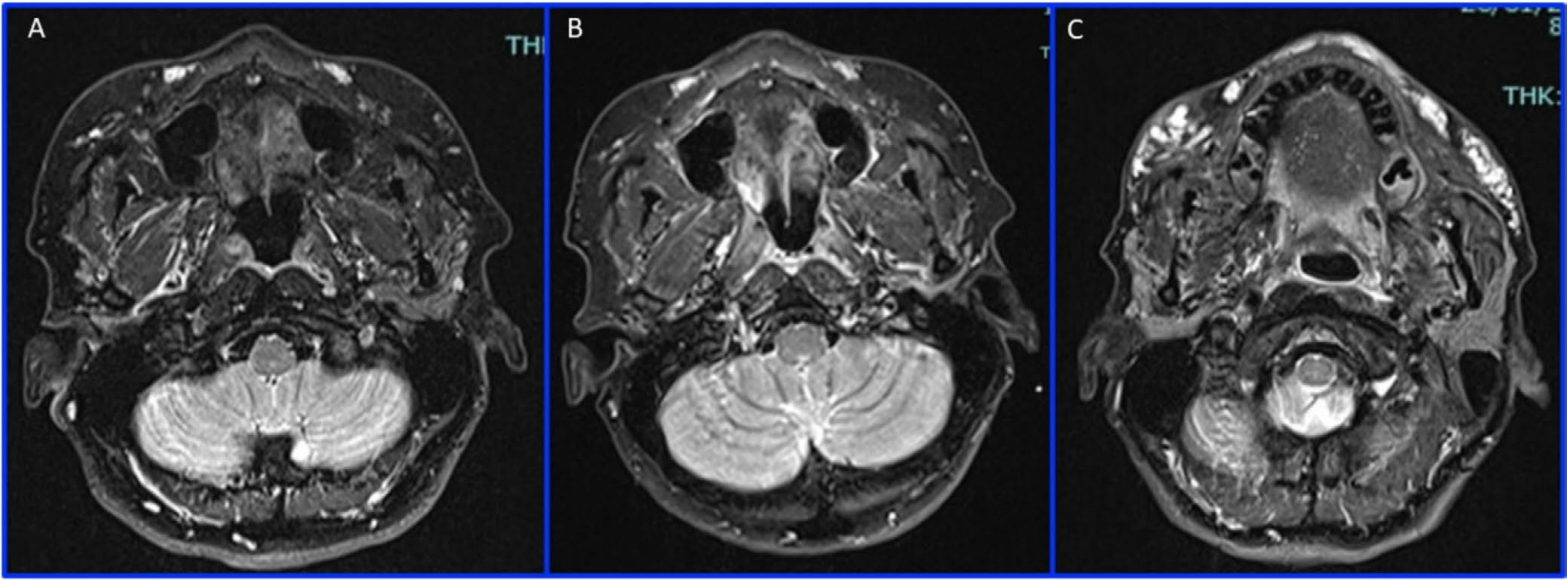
MRI: Axial T2 TIRM in I (A), II (B), e III (C) MRI, showing the persistence of HA filler in the pyriform recess 24 months after the first treatment.
